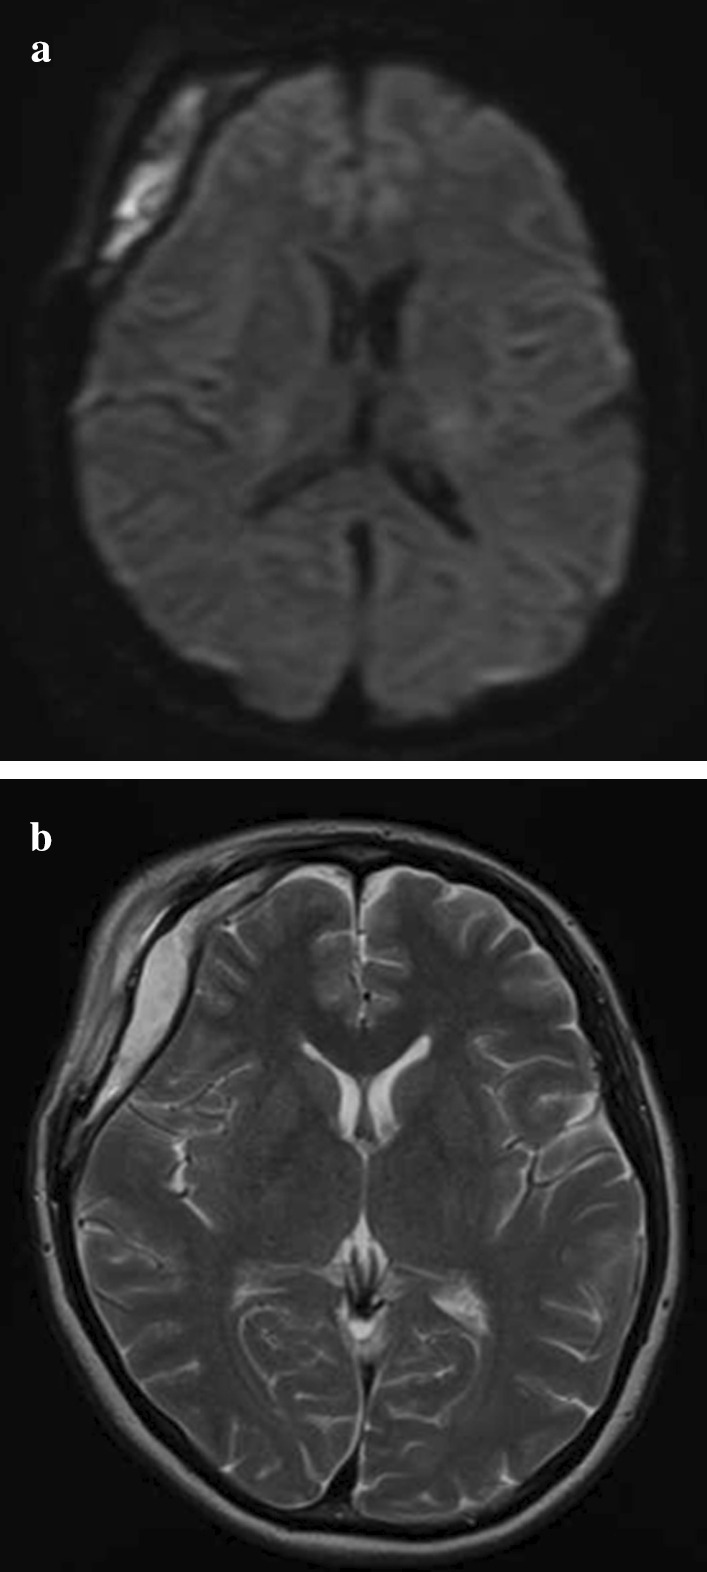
MRI of the first empyema. a Diffusion-weighted image. b T2-weighted image. The right frontal epidural area showed a high-intensity lesion on T2-weighted imaging and DWI
